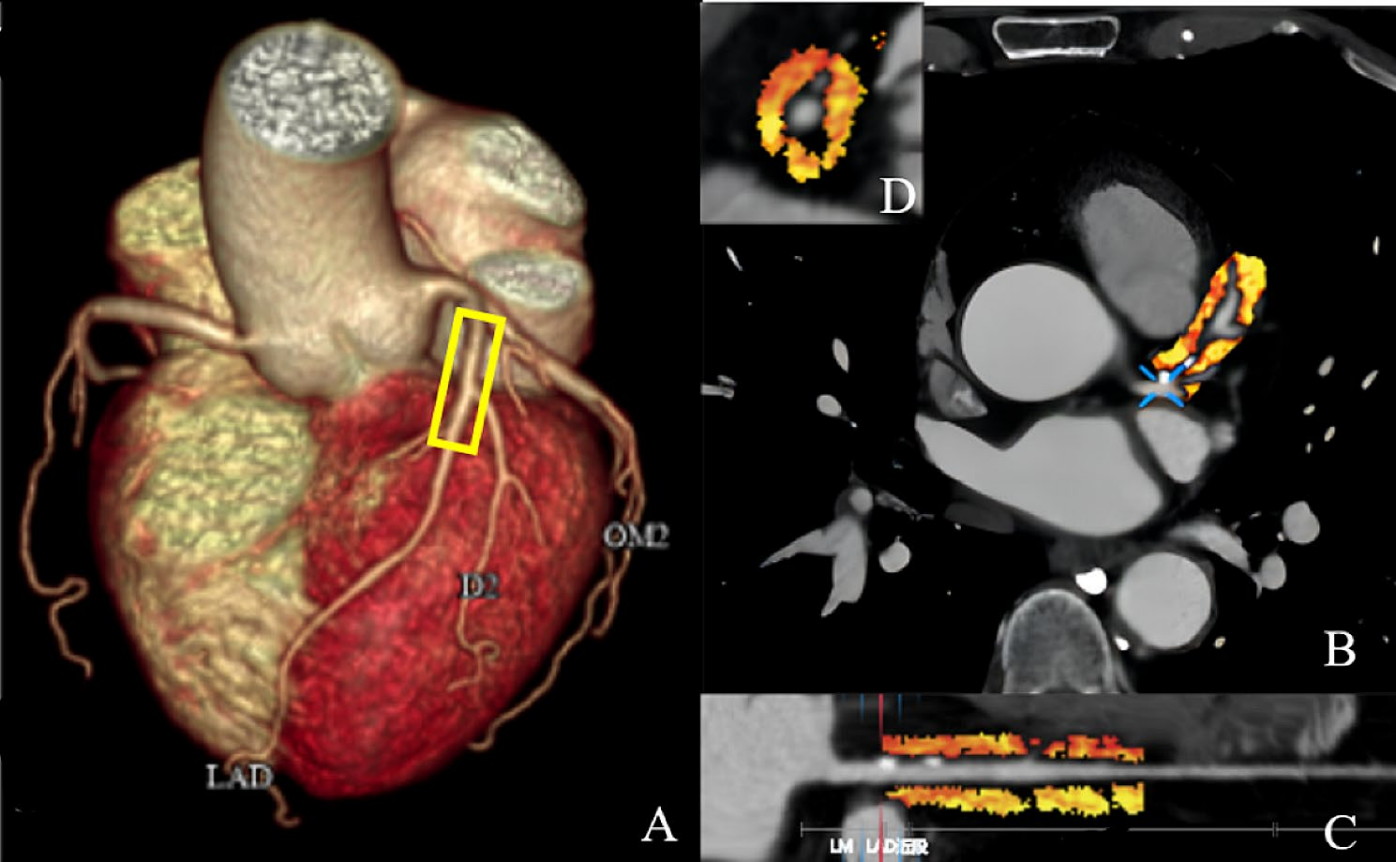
Representative case of LAD-PCAT attenuation measured by CCTA. A Three-dimensional reconstruction of the heart ; B PCAT attenuation between − 190 and – 30 HU in the cross-sectional view ; C The segment of the proximal coronary artery in a straightened view ; D Around the proximal 40 mm of the left anterior descending artery. *LAD* left anterior descending artery, *CCTA* coronary computed tomography angiography, *PCAT* Pericoronary adipose tissue
